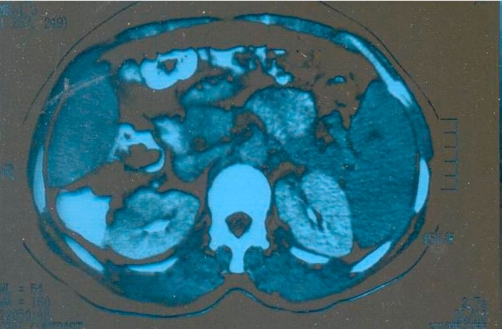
A pre-operative CT scan study showing normal right and left kidneys as well as pancreatic and para-aortic regions.
